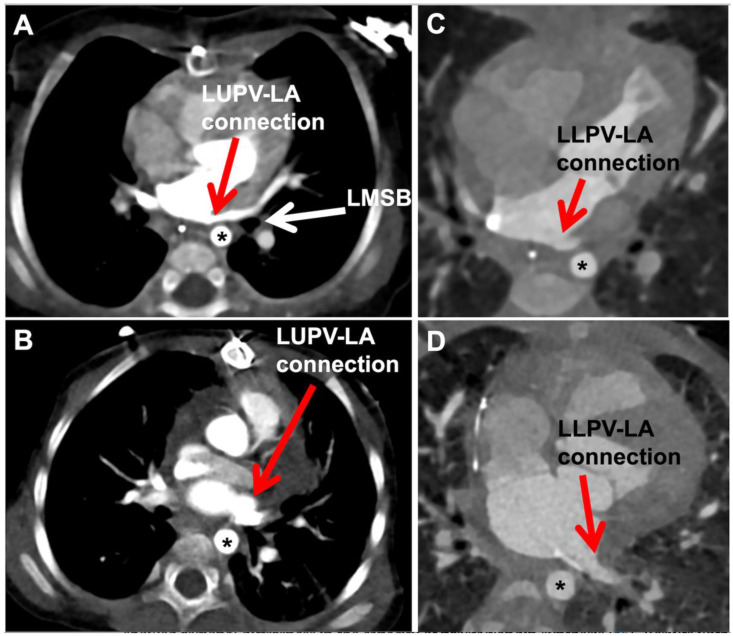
Pre- and post-repair pulmonary vein CT imaging. (A) Pre-operative left upper pulmonary vein taking long course over left bronchus before entering medially into left atrium; (B) Post-repair left upper vein course is shorter and more lateral—minimizing angulation; (C) Pre-operative left lower vein entering left atrium very medially after coursing over descending aorta; (D) Post-repair left lower vein takes shorter/straighter course as it enters atrium more laterally. (LUPV-LA = left upper pulmonary vein-left atrium; LMSB = left mainstem bronchus; LLPV-LA = left lower pulmonary vein-left atrium; Asterisk = descending thoracic aorta).
